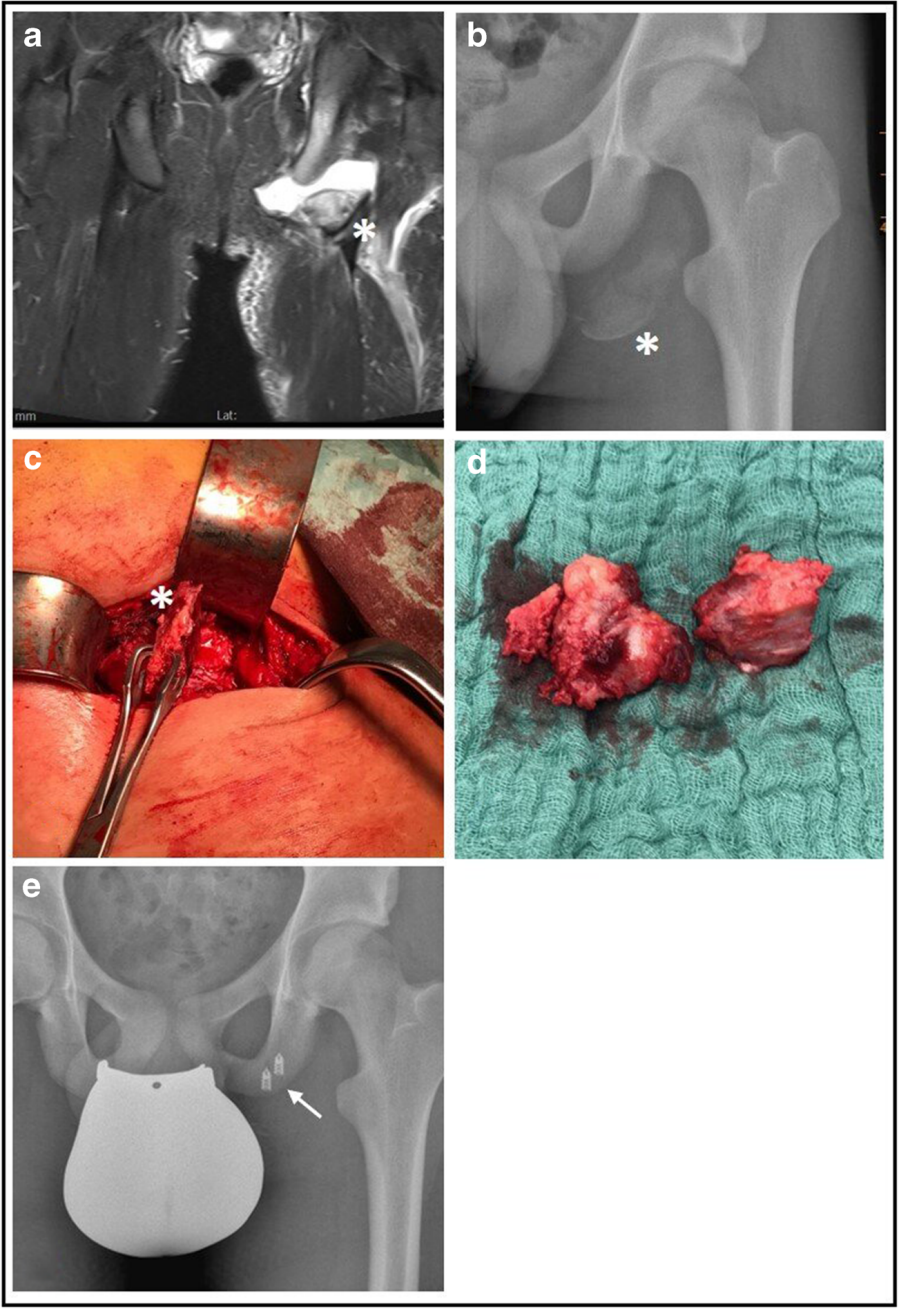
Excision of bony fragment in an acute left-sided avulsion fracture three weeks after trauma (a: coronal MRI picture, b: plain semipelvis radiograph, c: intra-operatively dissected bony fragment, d:excised bony fragment, e: post-operative plain radiograph control with refixated hamstring tendon, star*: bony fragment, arrow →: corkscrew anchors)
